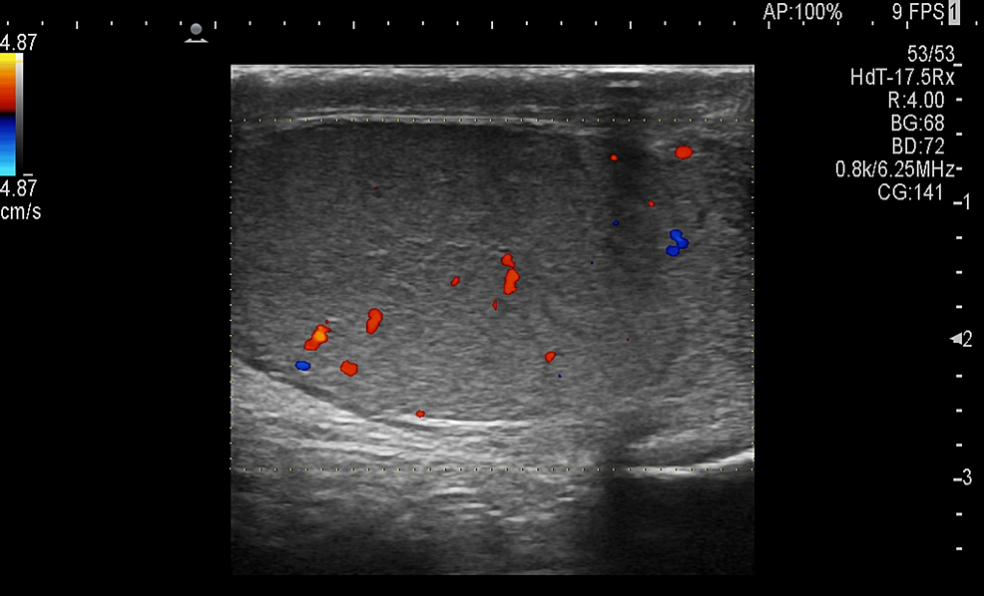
Ultrasound image showing normal appearance of the left testis with normal vascularity on color flow
